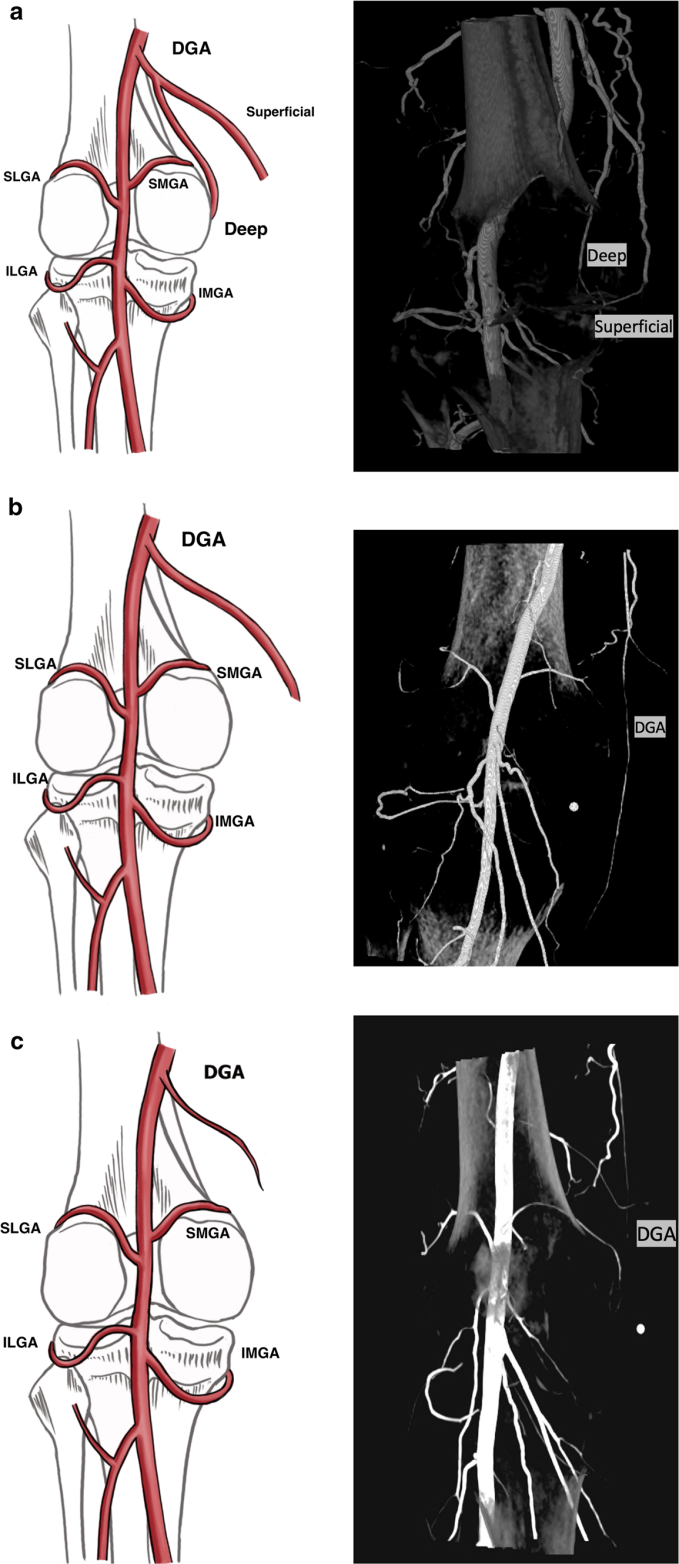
Descending genicular artery branching variants with intraoperative contrast-enhanced cone-beam computed tomography correlate. Four branching patterns were identified for the descending genicular artery (DGA), including a two branches (77%), b no branching (17%), c diminutive (5%), and absent (1%)
